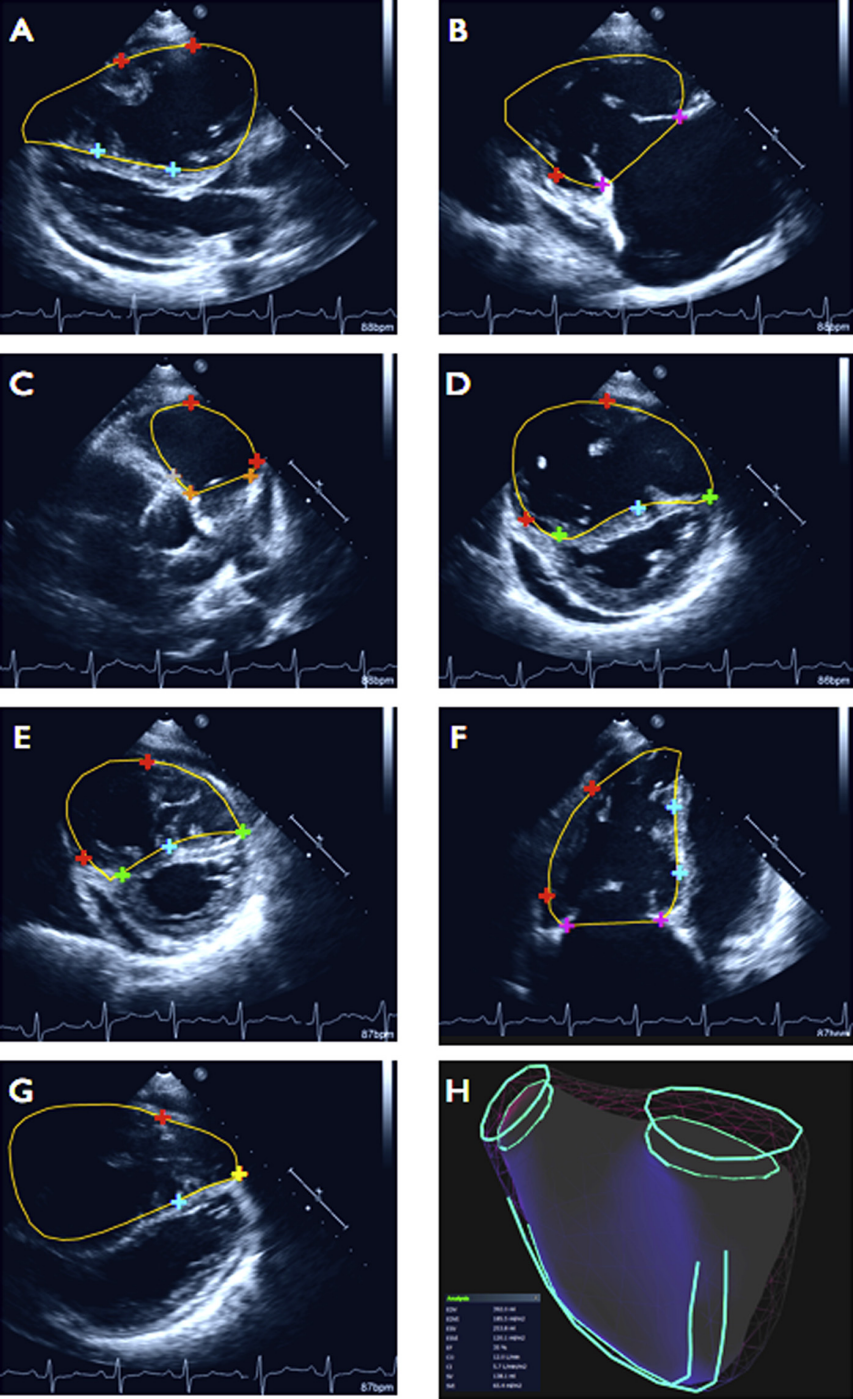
Postprocessed 2D KBR data from a participant with pulmonary hypertension. All of the required 2D echocardiographic scan planes in end-diastole are displayed: (A) parasternal long-axis (PLAX), (B) PLAX RV inflow, (C) PLAX RV outflow including infundibulum and pulmonary valve hinge points, (D) parasternal short-axis (PSAX) at midcavity (papillary muscle) level, (E) PSAX apical level, (F) four-chamber RV, (G) off-axis RV apical view (note how the RV apex rides over the left ventricular apex). The *differently colored cross-hairs* represent user-defined plots for different RV structures; for example, *red crosses* are plotted along the RV endocardium, *turquoise crosses* along the RV side of the interventricular septum, a *yellow cross* at the RV apex, *orange crosses* at the pulmonary valve annulus, and *purple crosses* at the tricuspid valve annulus. The *yellow border tracings* are superimposed projections of the 2D KBR RV reconstruction onto the original 2D echocardiographic scan data, also showing how the polygon extends beyond the original 2D echocardiographic image sector. Landmarks can be checked and repositioned by the user if required, and the 2D KBR algorithm subsequently rerun. A final check is the nested view (H) of end-diastolic and end-systolic polygons to ensure alignment of the tricuspid and pulmonary valve orifices.
